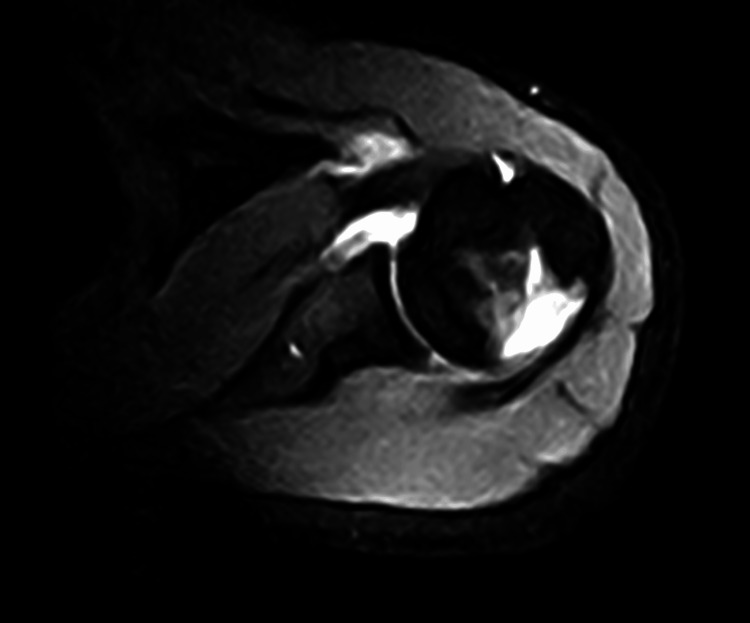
Pretreatment magnetic resonance imaging (MRI) of the shoulder. The initial scan reveals a consolidating Hill-Sachs lesion on the posterolateral surface of the humeral head, indicative of structural damage and associated inflammation.
